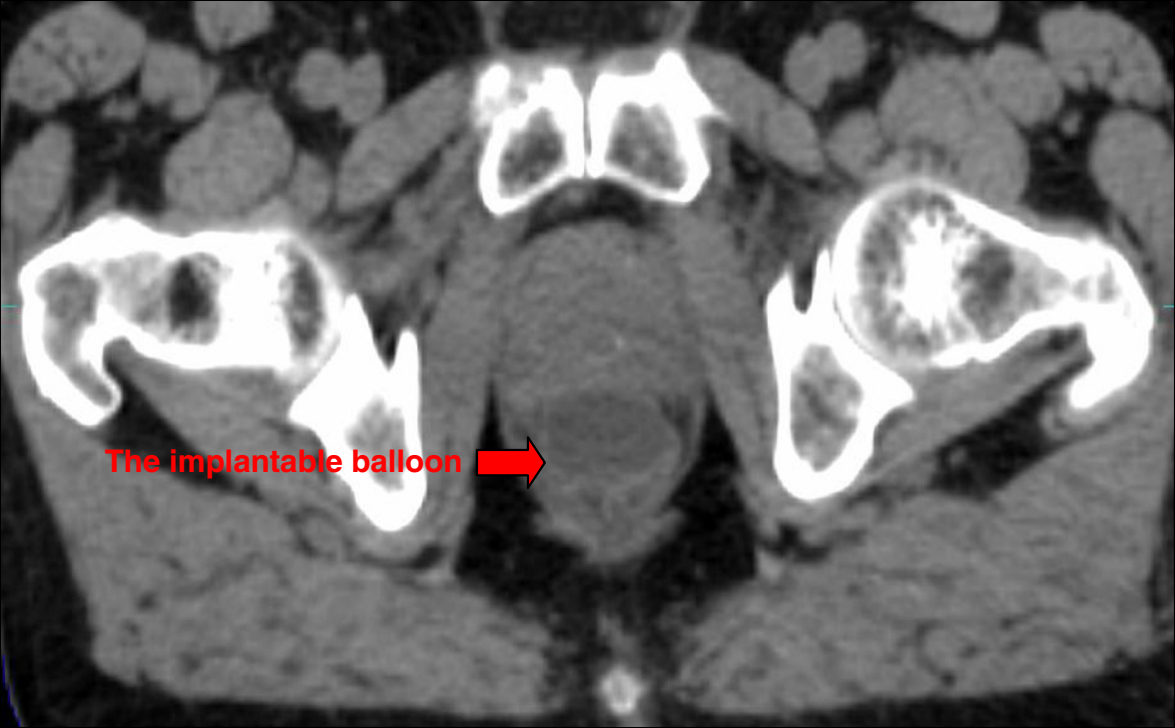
Axial view of CT scan, 7 days post balloon implant.
